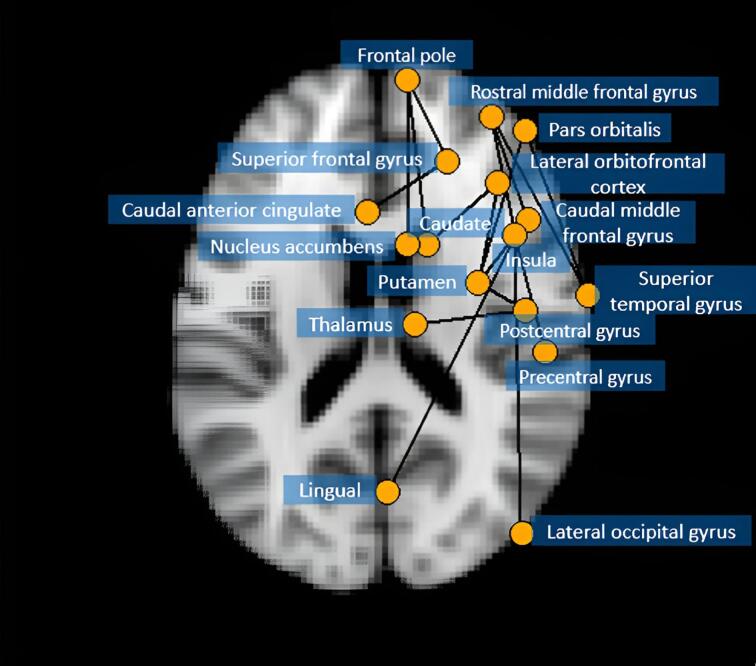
The NBS model projected onto a transverse MRI plane. The results show the network in which FA values were related to language performance.
